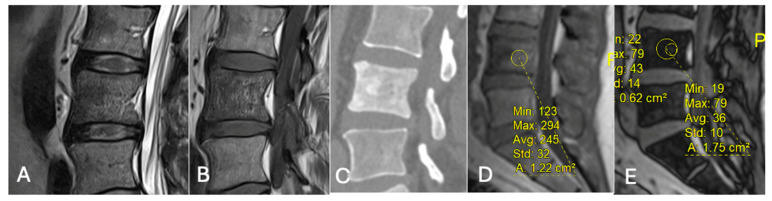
Focal marrow hyperplasia in L4. Sagittal T1 (A), T2 (B), CT (C), and chemical shift (D,E), showing heterogeneous signal on T1 and T2 and sclerosis on CT with significant signal drop on chemical shift images (D,E).
